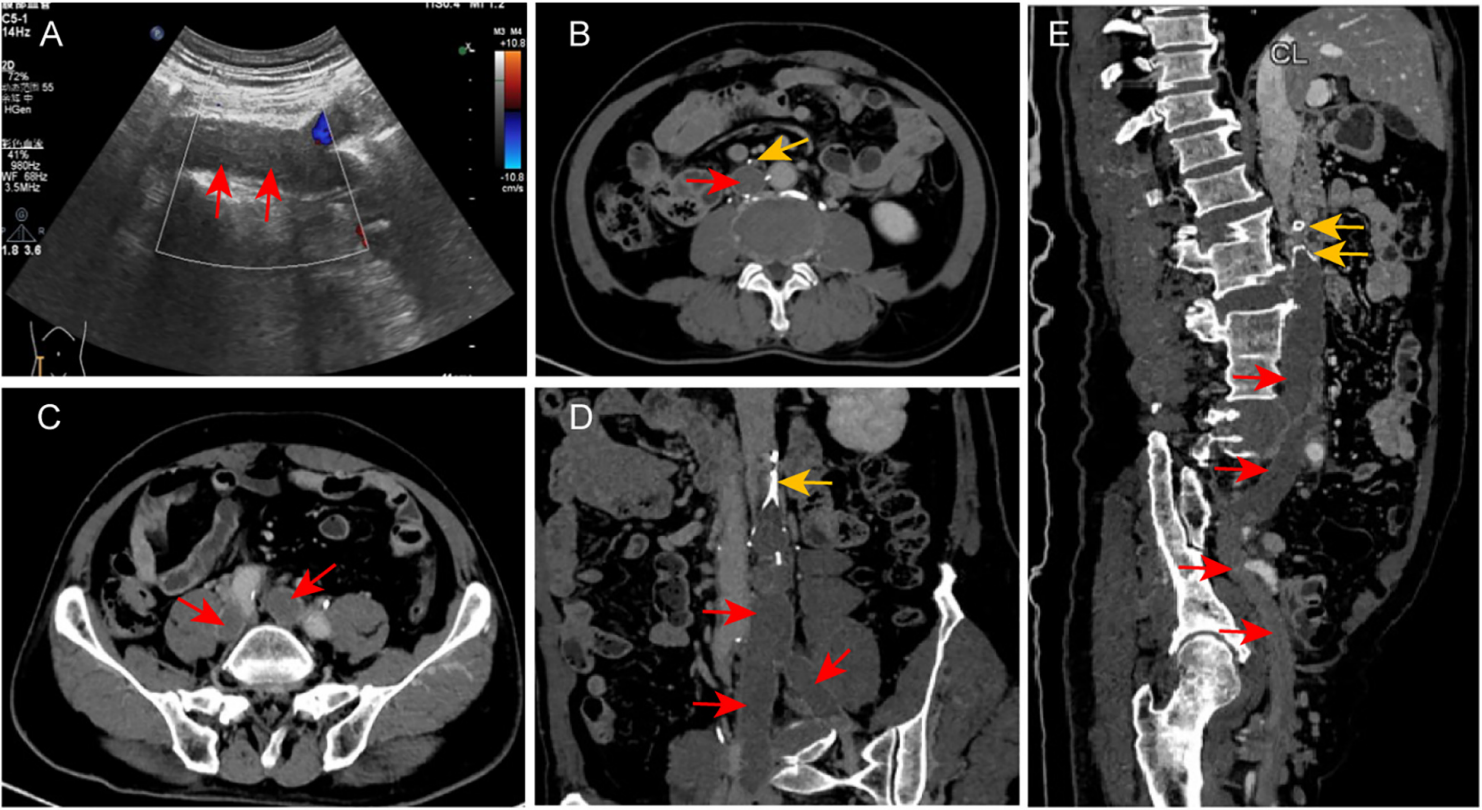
Imaging findings on admission. (A) Abdominal vascular ultrasound demonstrates hypoechoic thrombus (red arrows) in the IVC and bilateral iliac veins with absent Doppler flow signals, consistent with thrombosis. (B–E) CTV reveals: The implantation status of the patient's IVCF. The filter head and the retrieval hook are attached to the wall. There are extensive low-density shadows in the IVC below the filter, and the bilateral common iliac and external iliac veins, indicating extensive thrombosis (the red arrow points to the thrombus, and the yellow arrow points to the filter and its leg branches).
